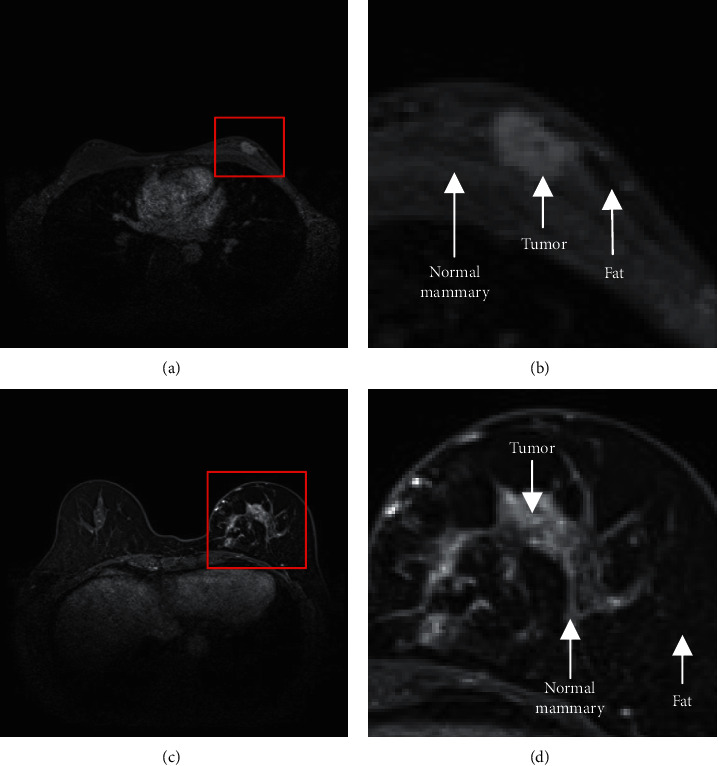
A mass and nonmass DCE-MRI breast tumor. (a) Mass breast tumor. (b) A partially enlarged view of the mass breast tumor. (c) Nonmass breast tumor. (d) A partially enlarged view of the nonmass breast tumor.
